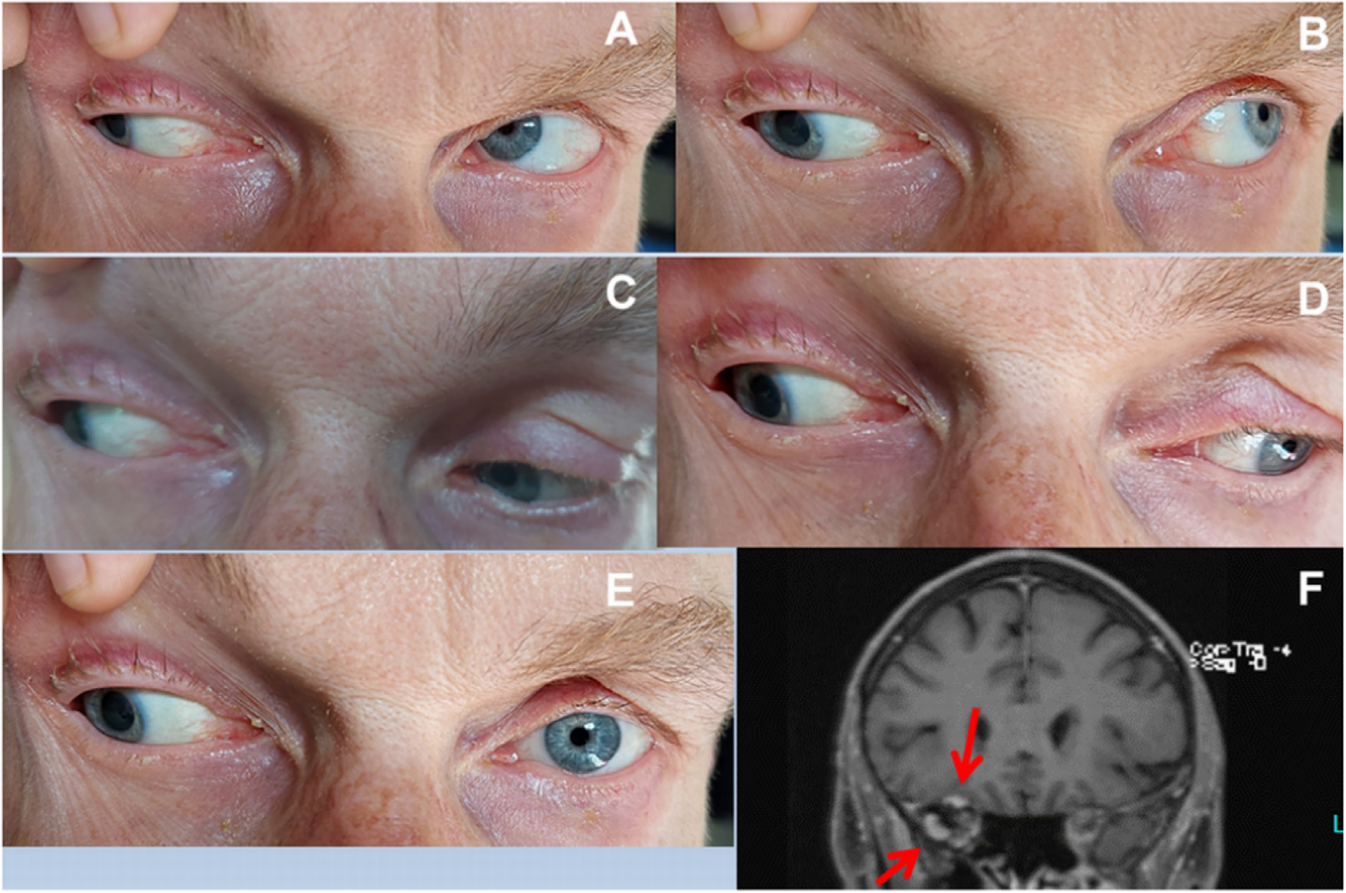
Right third cranial nerve palsy in the index patient (A: Gaze to the top right; B: Gaze to the top left; C: Gaze to the bottom right; D: Gaze to the bottom left, E: Gaze straight forward, F: Coronary T1-weighted image with contrast showing enhancement of the right superior lateral rectus muscles).
